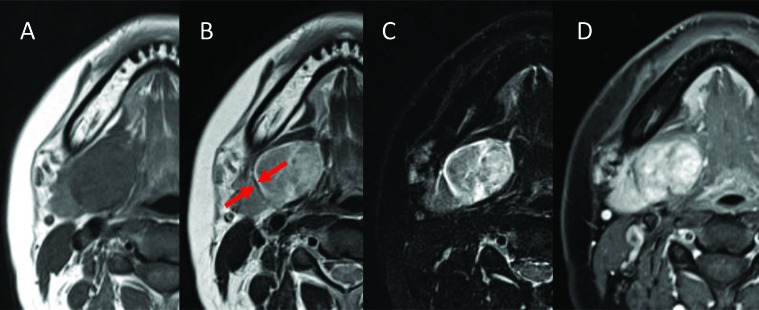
MR images showed homogeneous isointensity on T1-WI (A), heterogeneous hypointensity on T2-WI (B), heterogeneous hyperintensity on STIR (C), and heterogeneous enhancement on contrast-enhanced T1-WI (D). A clear capsule was observed on the margin (arrow).
